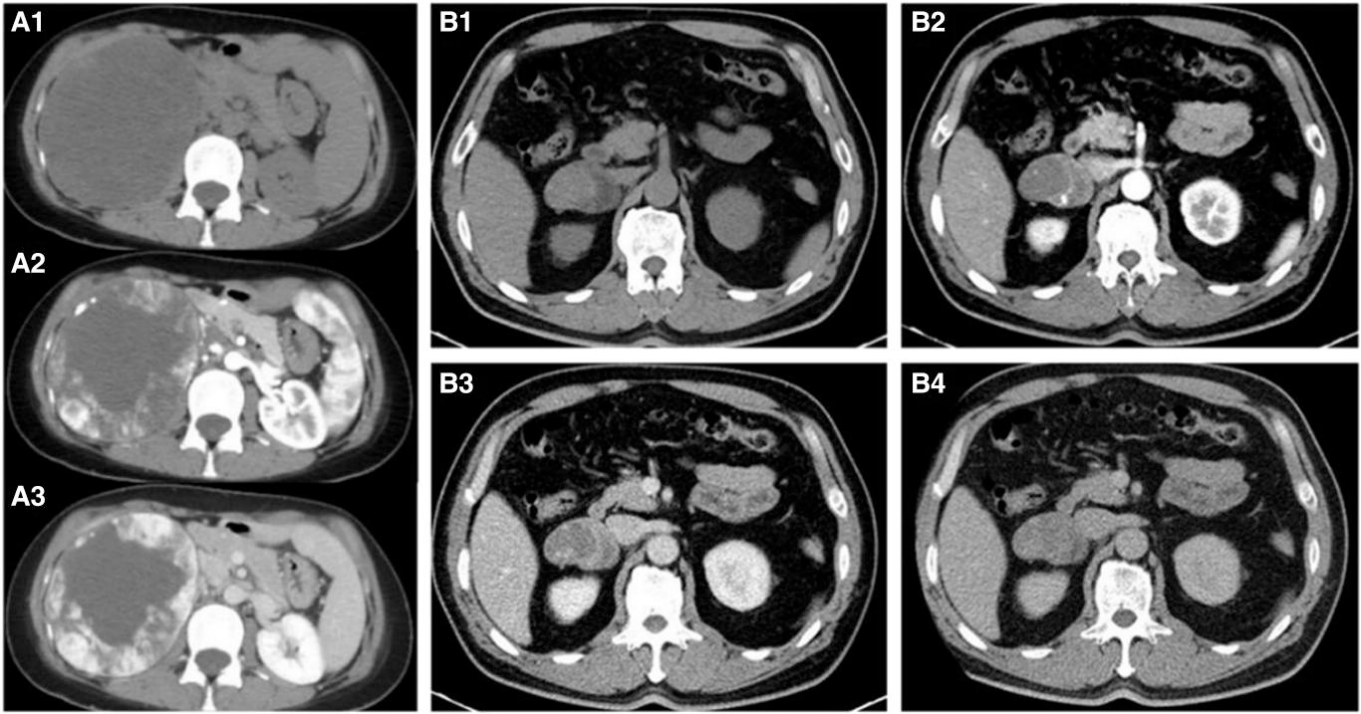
Collision tumors involving adrenal hemangioma. (A) Contrast-enhanced CT of a pheochromocytoma-hemangioma collision tumor. (A1-A3) shows typical centripetal progressive enhancement and central necrosis in the hemangioma combined with adrenal pheochromocytoma. (B) CT images demonstrating a hemangioma-adenoma collision tumor with distinct enhancement patterns. (B1) shows a higher density on the right side of the tumor on plain CT. (B2-B4) shows different enhancement features of the left and right sides of the tumor. The right side shows central progressive partial filling in the venous and delayed phase, while the left side shows moderate enhancement. Abbreviations: CT, computed tomography.
